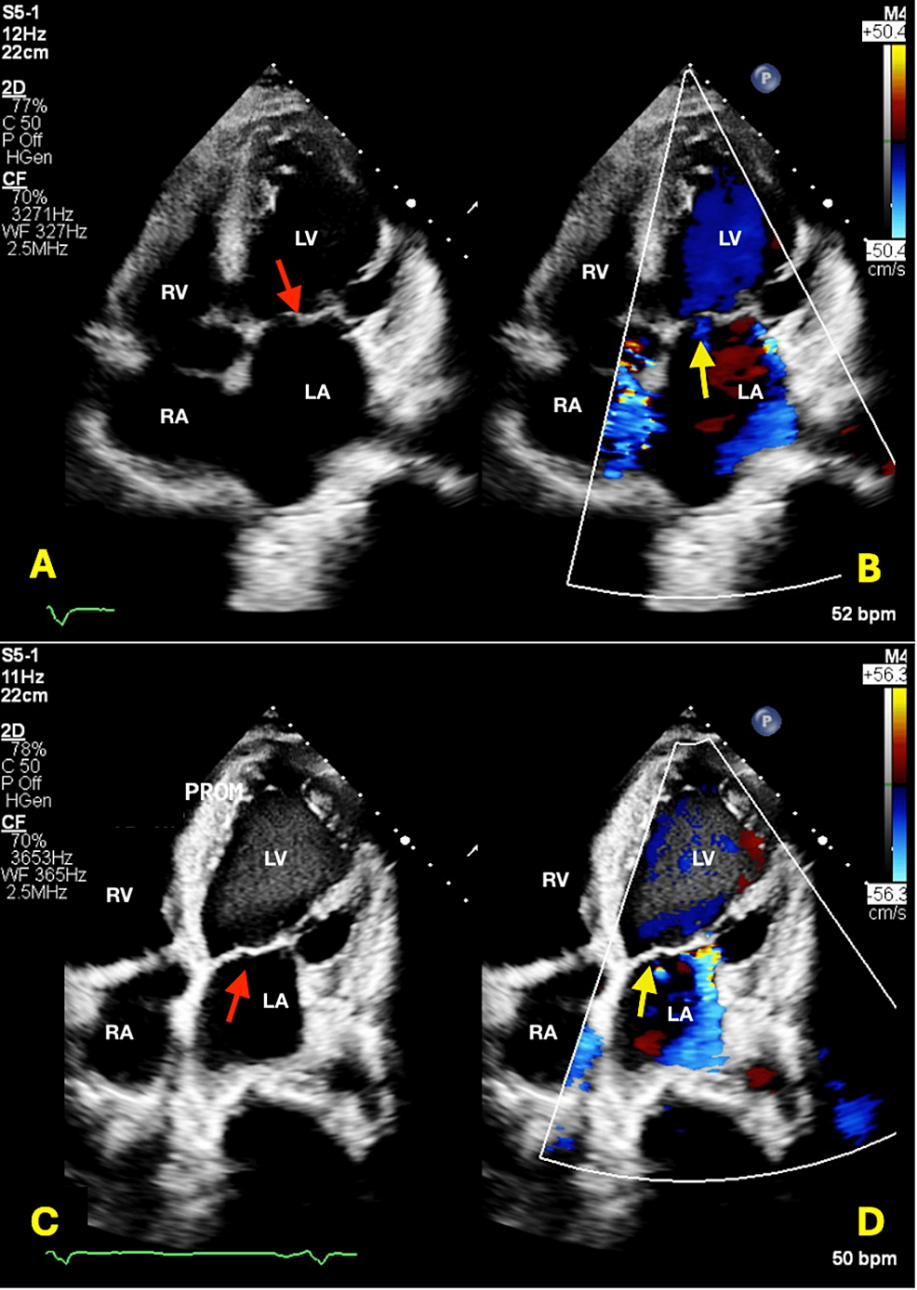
2D Transthoracic Echocardiogram: A & B: Akinetic heart, severe mitral regurgitation, ejection fraction of 25%; C & D: following mitral clip placement. Red arrows indicate valve pre and post mitral clip placement. Yellow arrows point to mitral valve with doppler flow. (B) shows mitral regurgitation and (D) indicates flow following mitral clip placement. RA: right atrium, RV: right ventricle, LA: left atrium, LV: left ventricle
